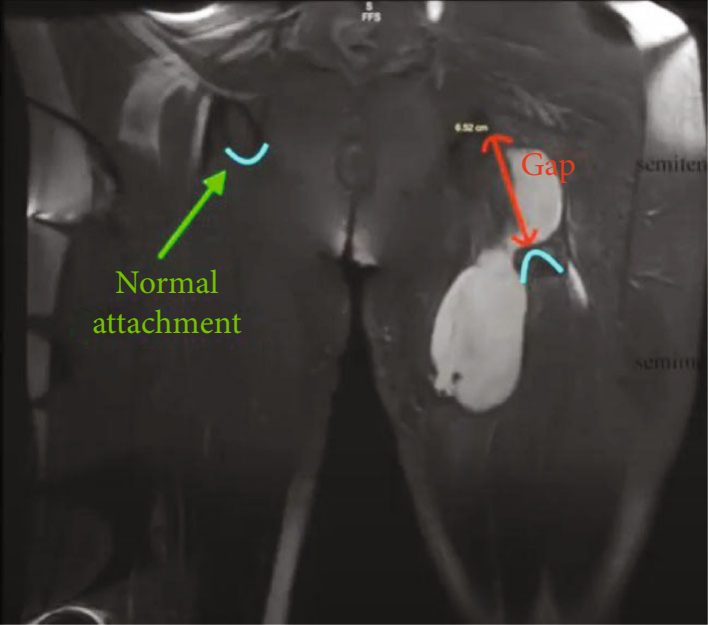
Coronal MRI displaying a retracted complete tear is of the left semitendinosus and biceps femoris tendons from the origin. Associated severe partial tear of the semimembranosus tendon with associated hematoma within the posterior deep musculature measuring up to 16.5 cm.
